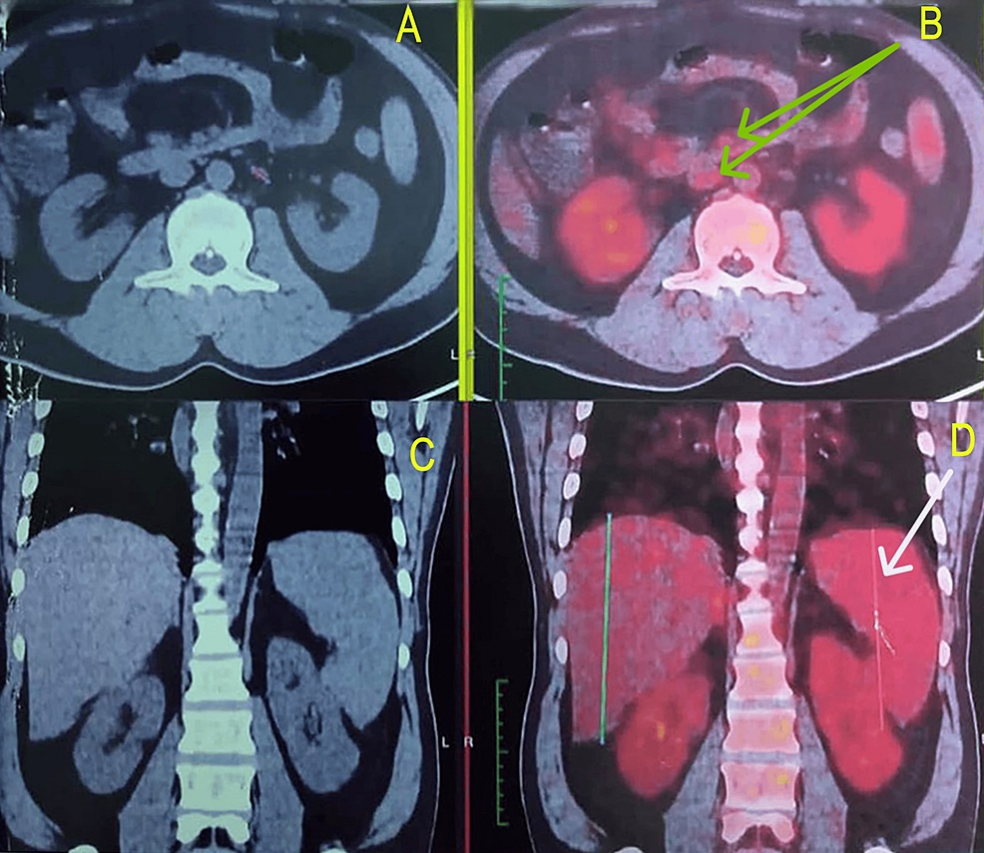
PET-CT scan showing hypermetabolic splenomegaly (see white arrow in image D), heterogenous increased bone marrow uptake, and few weakly metabolic left para-aortic nodes (see green arrows in image B). PET - positron emission tomography (see images B and D), CT - computed tomography (see images A and C)
